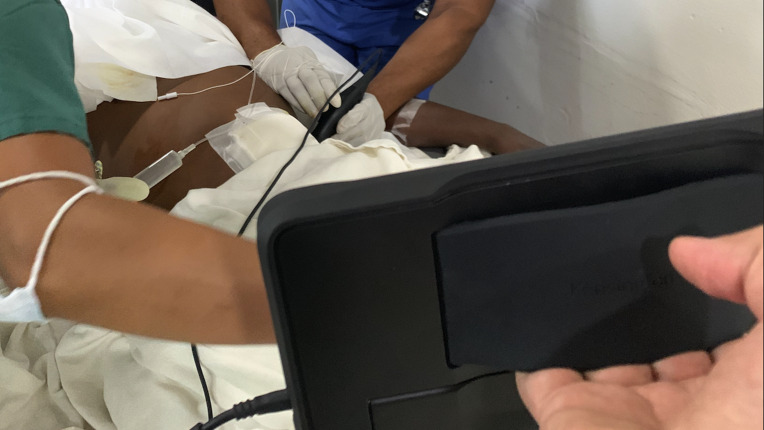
Post-operative use of point of care ultrasound (POCUS) for performance of Transversus Abdominis Plane (TAP) block.
